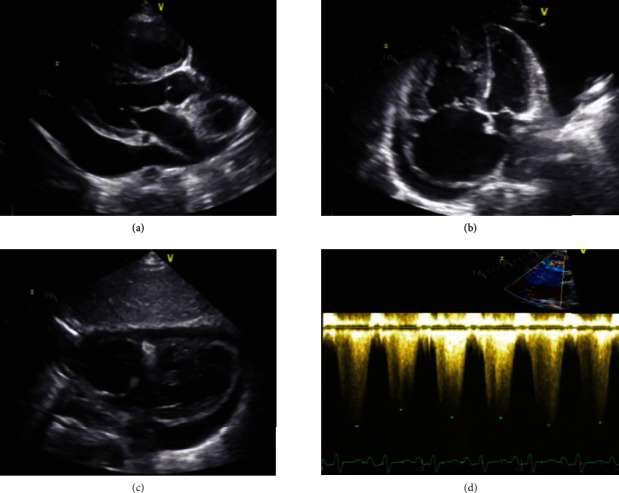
Admission transthoracic echocardiography showing circumferential pericardial effusion, reaching posteriorly 26 mm without compression of right atrium and ventricle. There is normal left ventricular wall thickness and contractility (LVEF > 70%). Severe right ventricular hypertrophy with flattened septum and severe PASP. There is a severe functional TR due to annulus dilatation and tethering of the tricuspid leaflets.
